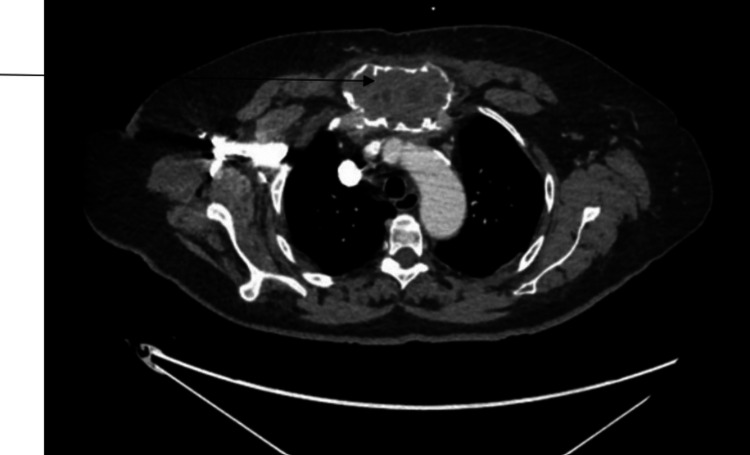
CT of the chest showing the manubrial expansile lesion. The arrow points to the expansile lesion in the CT scan image.
